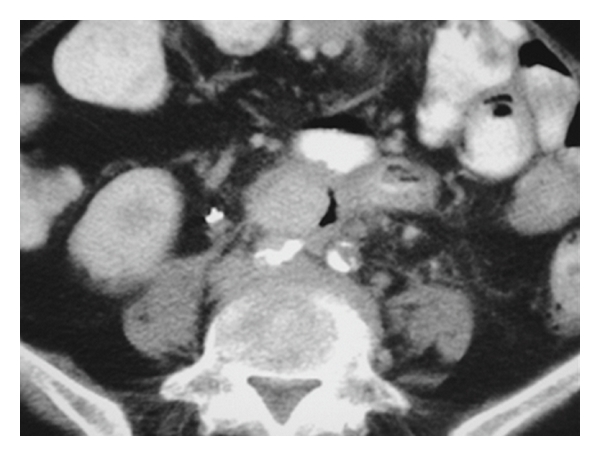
CT image of patient 5, who presented with unexplained fever. CT shows periaortic graft gas.
